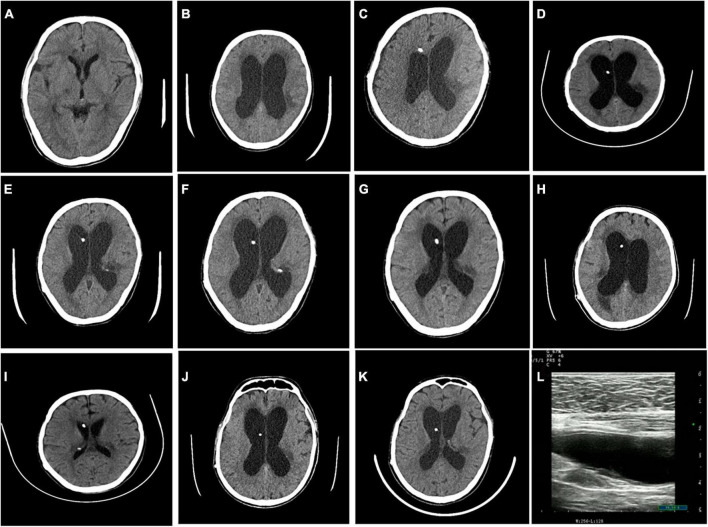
Cranial CT findings during the patient’s six hospitalizations. No hydrocephalus was observed during the first hospitalization (A). Hydrocephalus was visible before the operation during the second hospitalization (B), and hydrocephalus was relieved post-operatively (C). Hydrocephalus was visible before the operation during the third hospitalization (D), and hydrocephalus was relieved post-operatively (E). Hydrocephalus was visible before the operation during the fourth hospitalization (F), and hydrocephalus was relieved post-operatively (G). Hydrocephalus was visible before the operation during the fifth hospitalization (H), and hydrocephalus was relieved post-operatively (I). Hydrocephalus was visible before the operation during the sixth hospitalization (J), and hydrocephalus was relieved post-operatively (K). Abdominal ultrasonography before surgery suggested an intra-abdominal encapsulated effusion (L).
